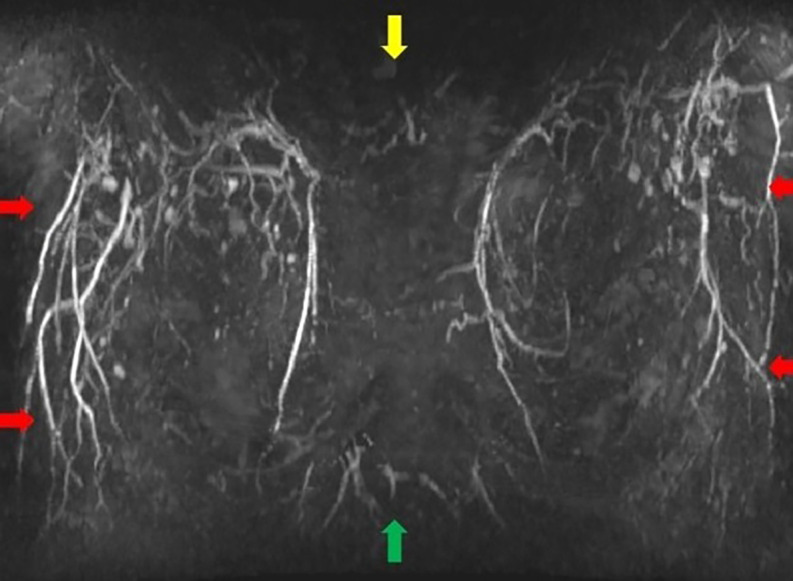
Coronal MRI maximum intensity projection (MIP) images. The manubrium of the sternum is at the top of the image (yellow arrow), and the xiphoid is at the bottom of the image (green arrow). The lateral mammary vessels are more dominant and robust than the perforators arising from the internal mammary vessels (red arrows).
